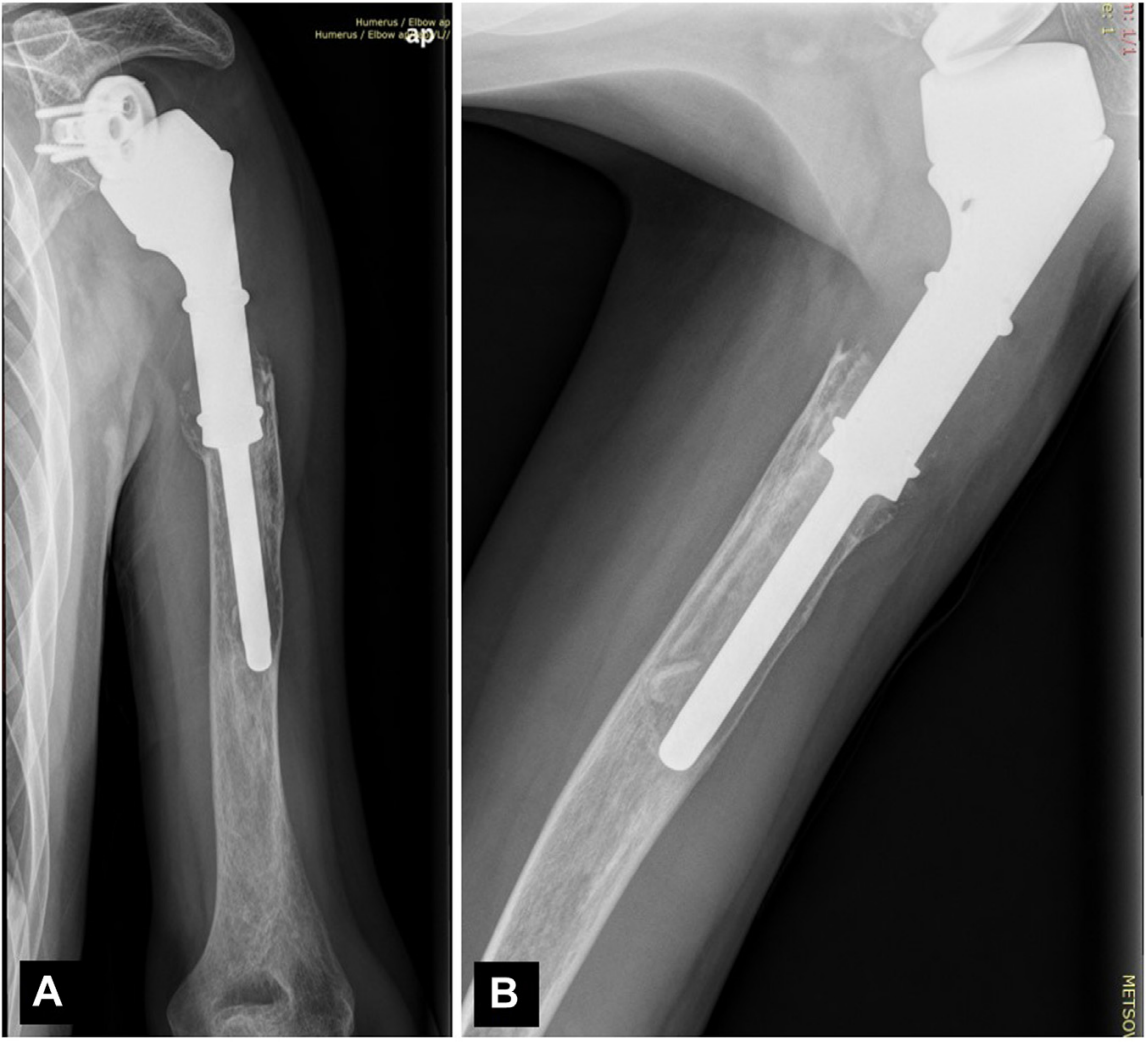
(A, B) Follow-up X-rays 2 years later, showing osteolysis of the proximal part of the humerus, loosening and extrusion of the prosthesis toward the lateral cortex.
